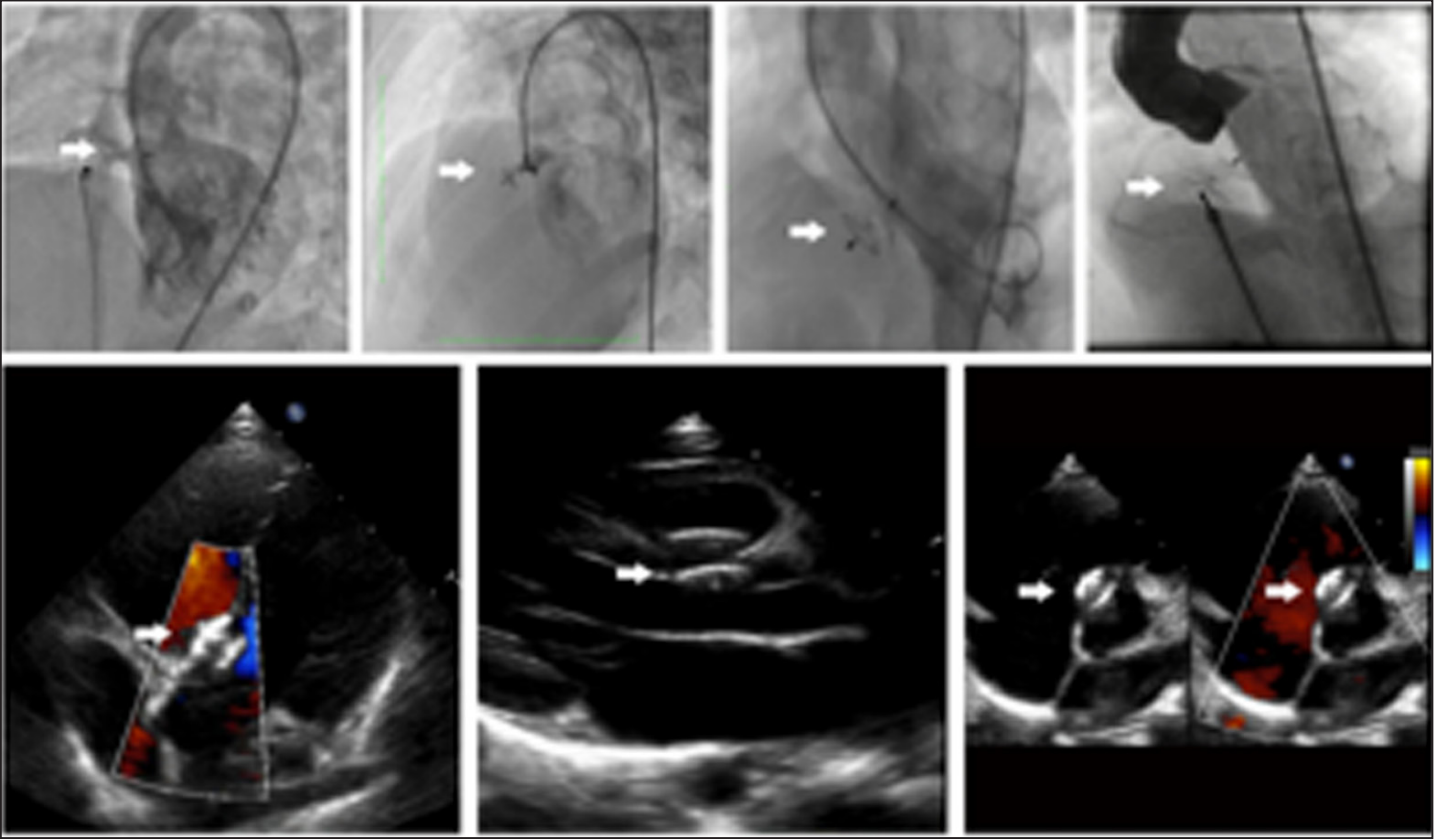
Angiography and echocardiography after closure.
